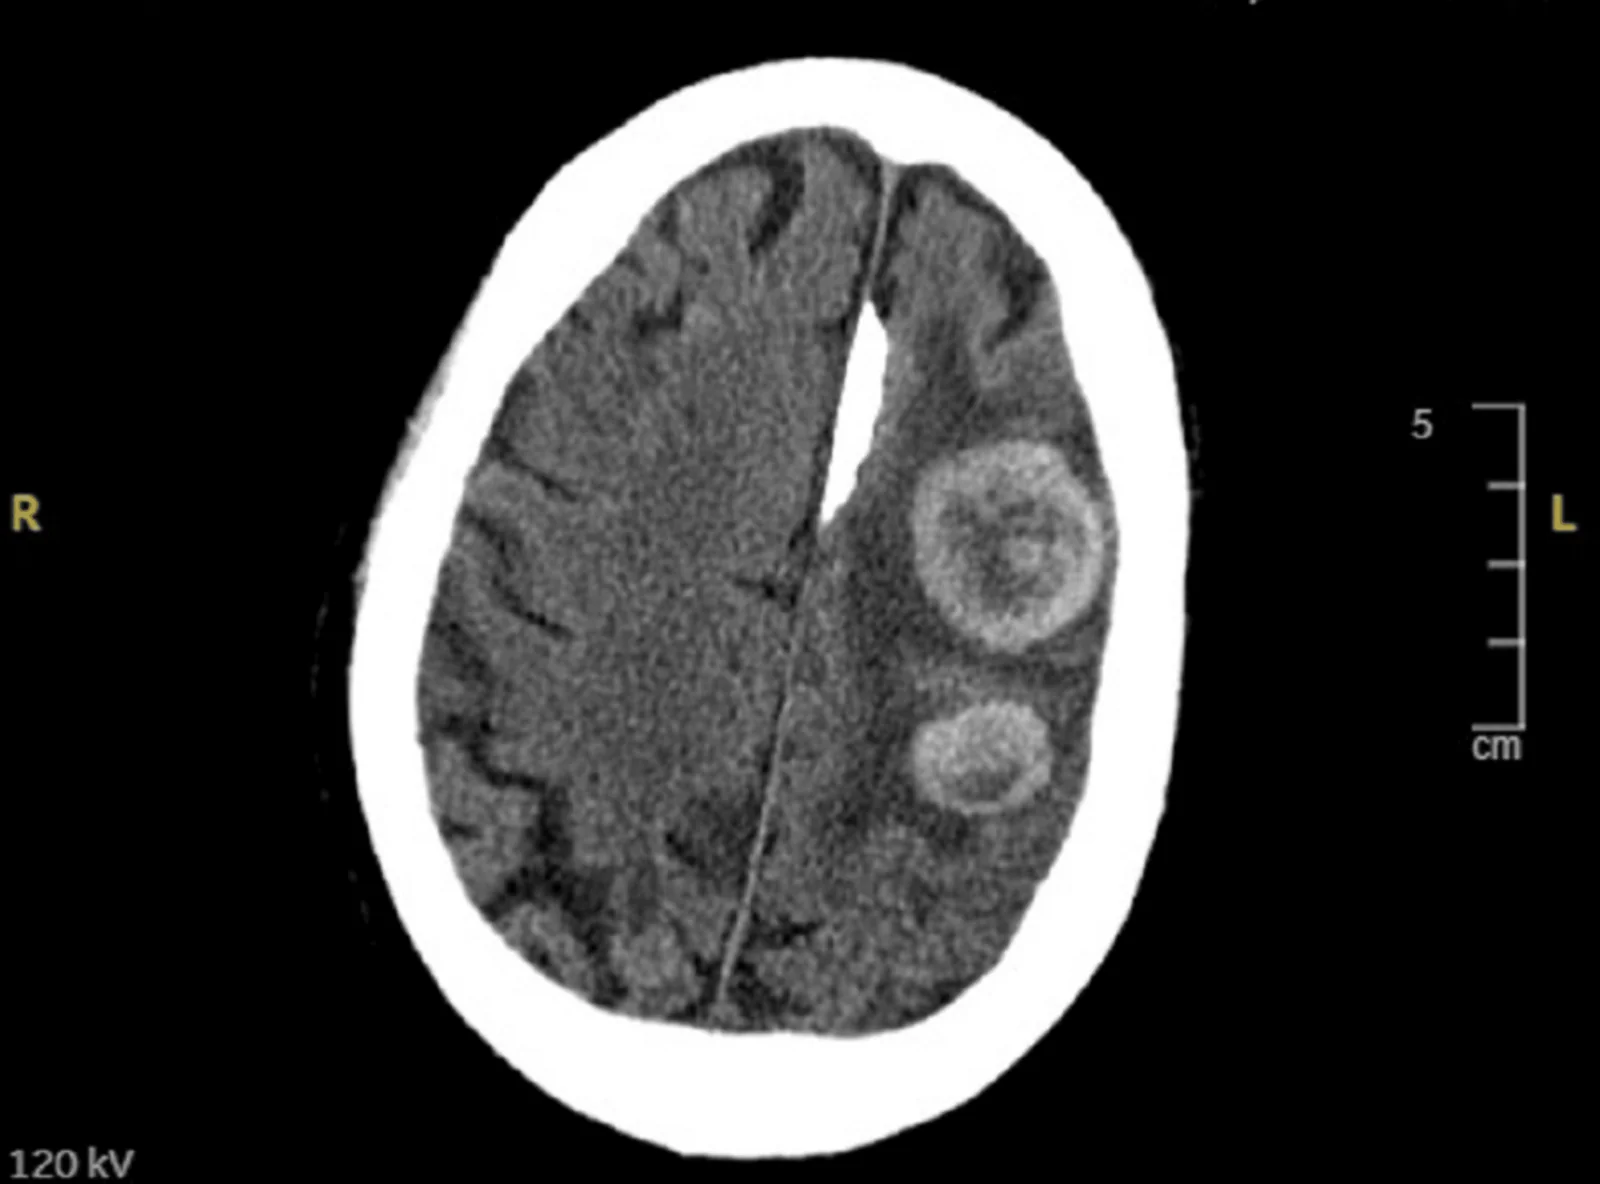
Head CT demonstrating left frontal lobe lesions with associated edema Axial CT of the head demonstrates left frontal lobe intra-axial lesions with associated perifocal edema. The largest lesion measures approximately 2.9 × 3.2 cm CT: computed tomography
